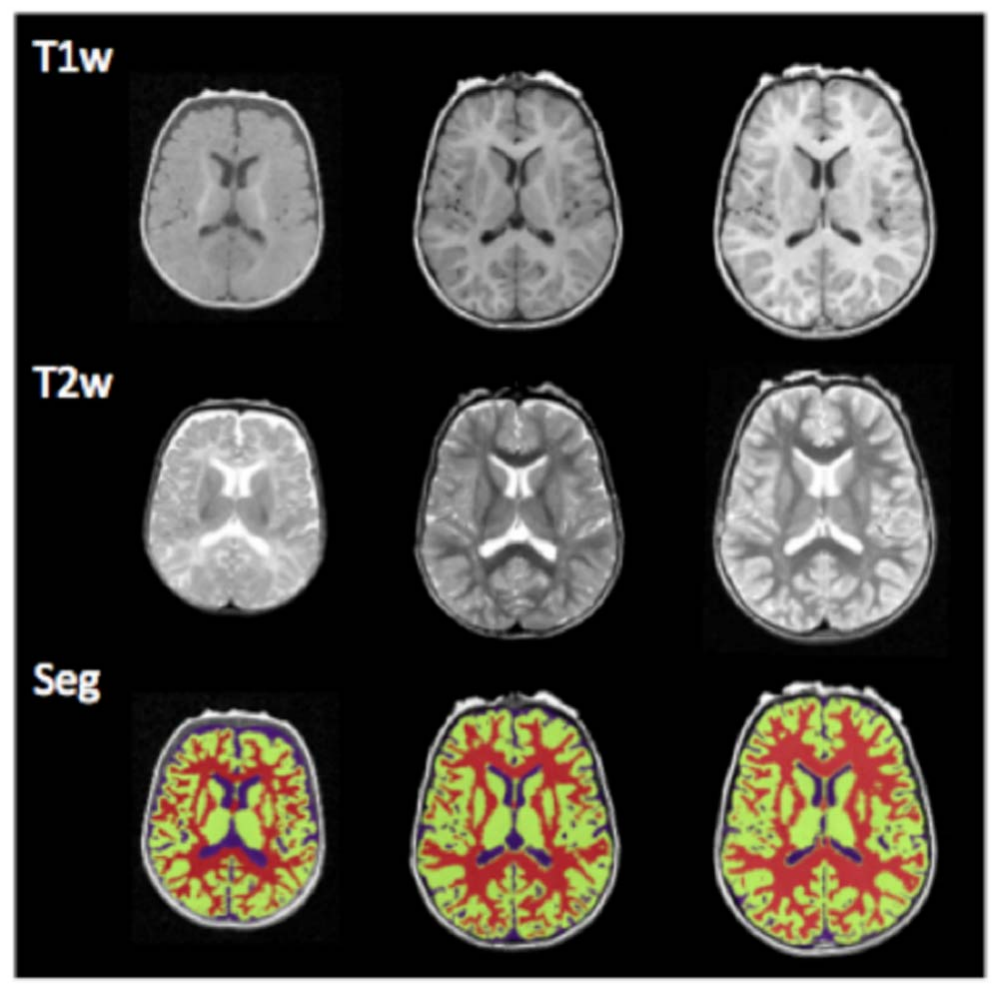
This figure shows example axial slices from a typical subject scanned at birth (left column), 2 (middle column), and 4 years old (right column). T1 MRI, T2 MRI, and segmented gray matter (green) and white matter (red) are provided.
